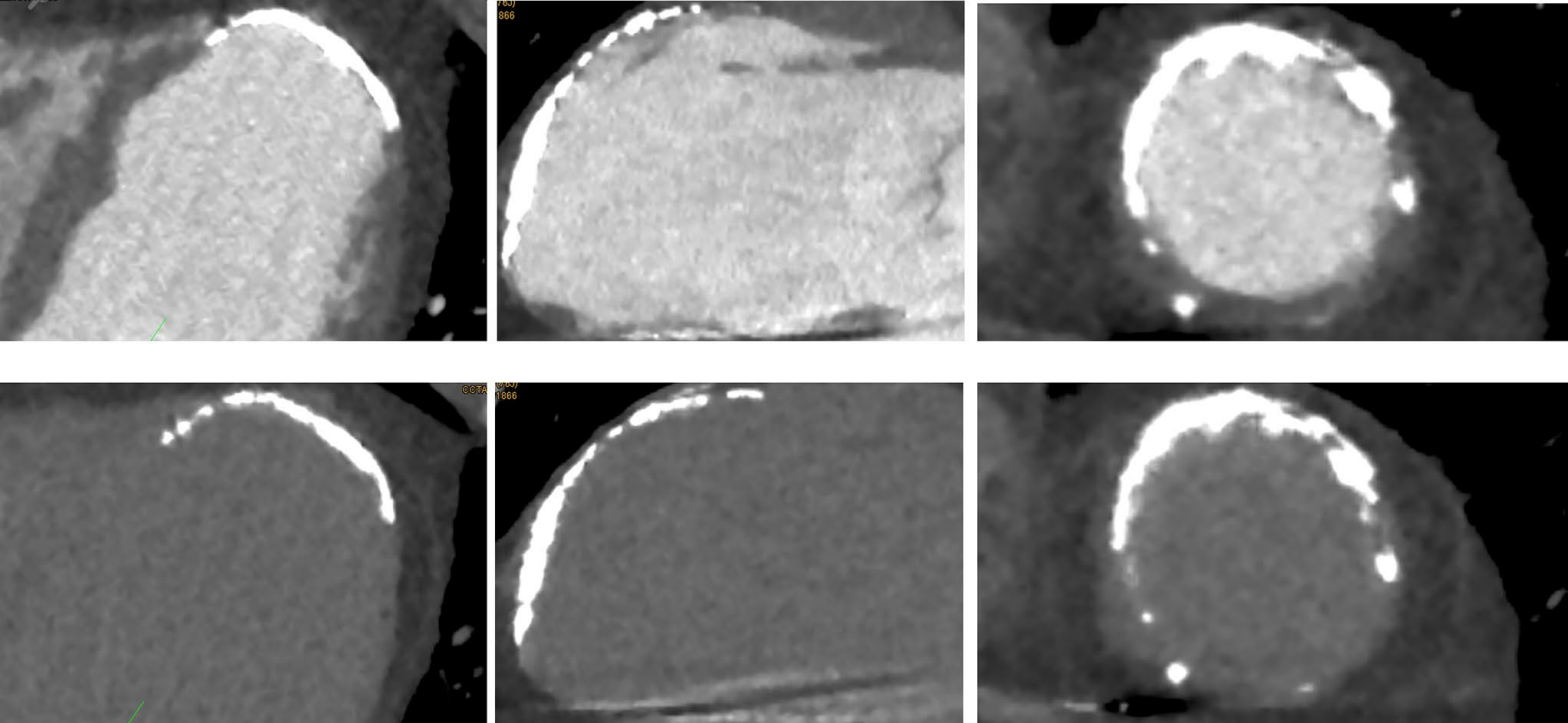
Use of CCT in the context of VT ablation. A 55-years old patient with ischemic cardiomyopathy and history of extensive myocardial infarction underwent a CCT for exclusion of LV thrombus in the LV. Upper panel demonstrates three chamber, two chamber and short axis CCT contrast enhanced images of the LV. Lower panel depicts the delayed phase imaging whereby a thrombus can be safely excluded based on the homogenous enhancement of the severely calcified, akinetic regions of the LV apex, the anterior and anteroseptal LV myocardium. The same contrast-enhanced CCT images were utilized peri-interventionally to guide the ablation procedure. LV; left ventricle, CCT; cardiac computed tomography, VT; ventricular tachycardia
